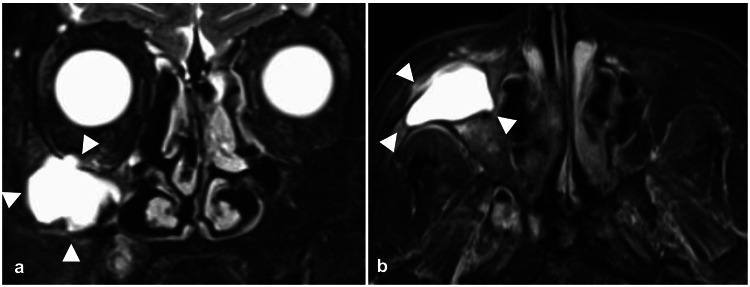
Preoperative magnetic resonance imaging (MRI) T2-weighted images are shown. A structure with a clear border from the surrounding area and a nearly homogeneous internal water signal was identified in the right maxilla and diagnosed as a postoperative maxillary cyst (POMC). White arrowheads indicate the POMC. (a) shows a coronal slice and (b) shows an axial slice.
